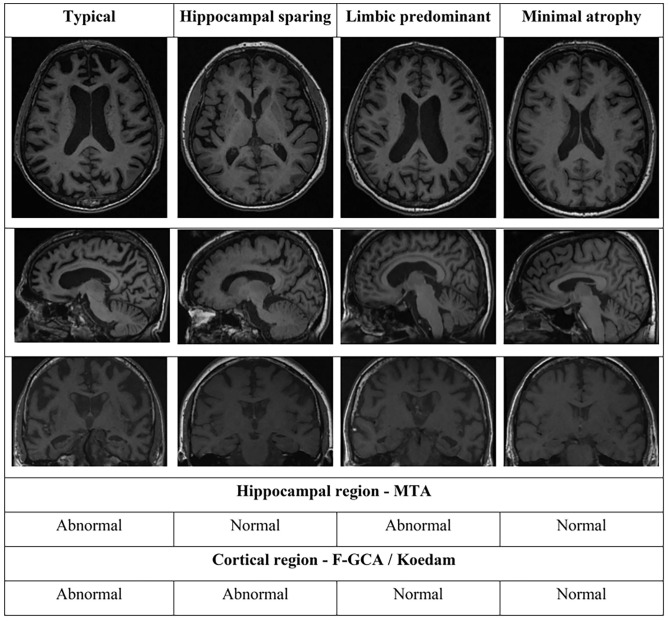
Representative T1-weighted MRI examples of the four scale-based phenotypes identified in our cohort. Columns (left to right) show the typical, hippocampal sparing, limbic predominant, and minimal atrophy phenotypes. For each representative case, axial 3D T1w images (top row), sagittal 3D T1w images (middle row), and paracoronal T1w images oriented parallel to the long axis of the hippocampus (bottom row) are shown. Phenotype classification was based on visual assessment of medial temporal atrophy (MTA), frontal cortical atrophy using the frontal subscore of the global cortical atrophy scale (F-GCA), and posterior atrophy (PA; Koedam scale). The typical phenotype was defined by pathological MTA together with pathological F-GCA and/or PA; hippocampal sparing by normal MTA with pathological F-GCA and/or PA; limbic predominant by pathological MTA with normal F-GCA and PA; and minimal atrophy by normal MTA, F-GCA, and PA. The same visual rating scales were used for both the standard scale-based and adjusted scale-based phenotyping approaches. All images were obtained from study participants and fully anonymised in accordance with the study ethical approval. Abbreviations: F-GCA, frontal subscore of global cortical atrophy; MRI, magnetic resonance imaging; MTA, medial temporal atrophy; PA, posterior atrophy; T1w, T1-weighted.
